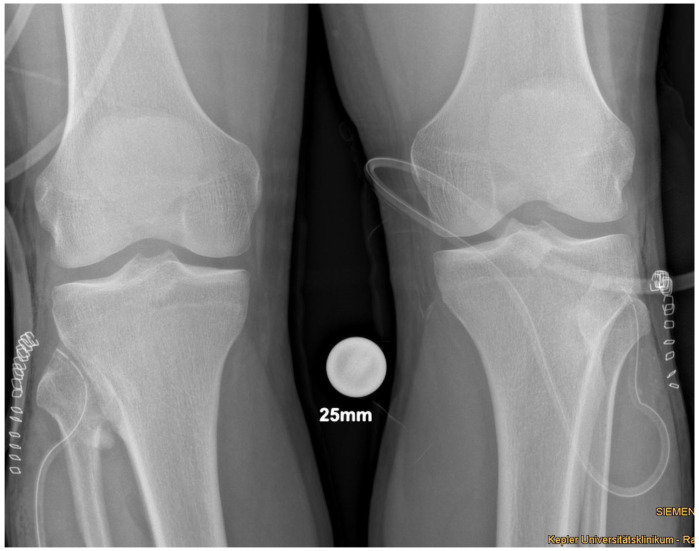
Plain radiograph in a.-p. view after resection.
